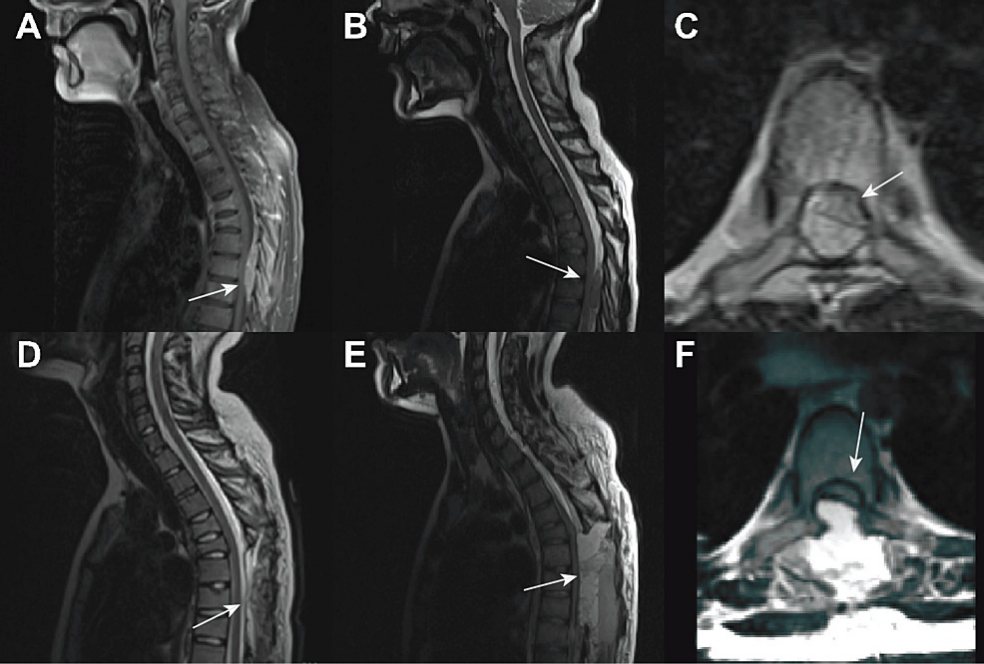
Pre and postoperative MRI A: Sagittal T1 with contrast MRI scan showing epidural intramedullary metastasis in the thoracic level six through eight; B: Sagittal T2 MRI scan showing epidural extramedullary metastasis in thoracic level six through eight; C: Axial T1 with contrast MRI scan showing thoracic epidural extramedullary metastasis D: Postoperative day 1 sagittal T2 MRI scan detailing the thoracic level six through eight laminectomies and decompression; E: Postoperative day 9 sagittal T2 MRI scan detailing the new acute onset thoracic epidural hematoma; F: Postoperative day 9 axial T2 MRI scan detailing the new acute onset thoracic epidural hematoma and notable thecal sac compression.
